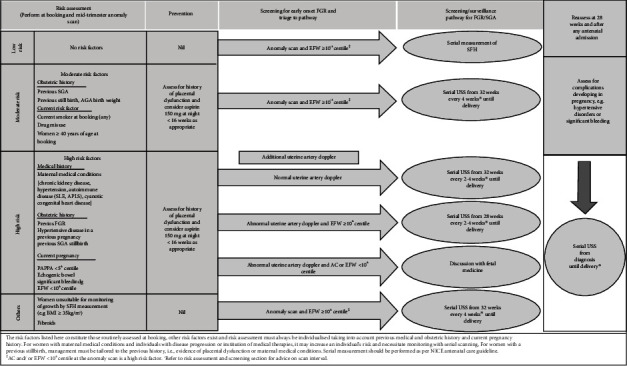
Algorithm describing uterine artery Doppler as a screening tool for risk of early-onset FGR [1].
